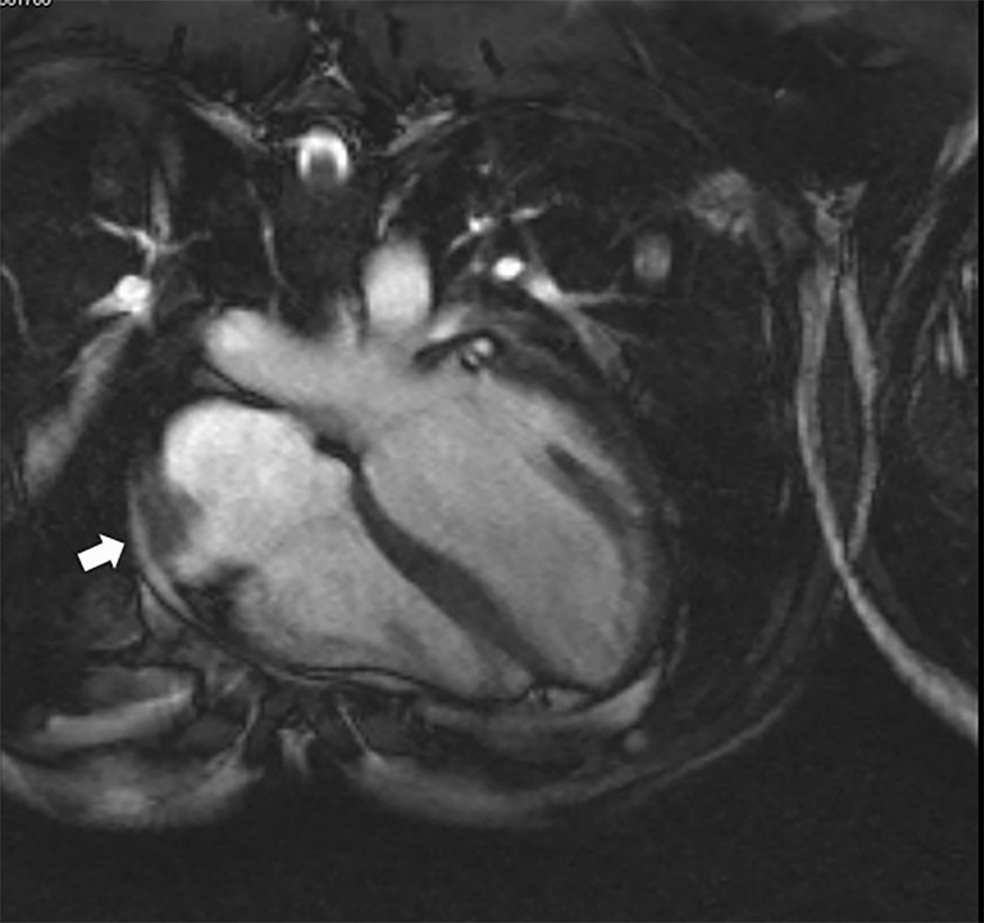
Cardiac MRI. Mass of 8 x 3.5 x 2 cm at the anterior wall of the right atrium (arrow).
